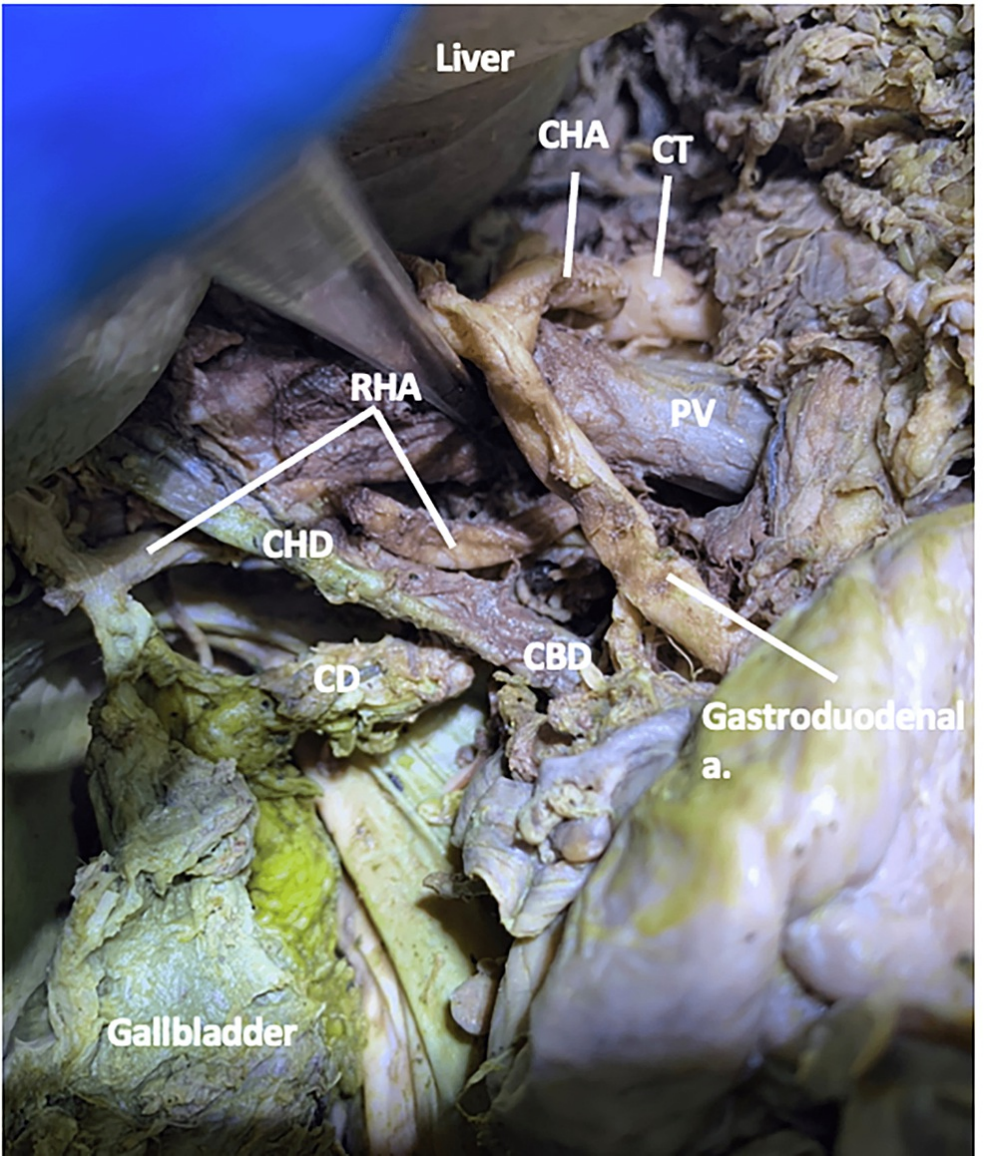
The spatial relationships of the right hepatic artery in case three Case 3: In a 90-year-old female, the right hepatic artery originated from the superior mesenteric artery and traveled deep to the pancreatic neck. The RHA coursed between the portal vein and the proper hepatic artery as it entered the cystic triangle. a: artery; CBD: common bile duct; CD: cystic duct; CHA: common hepatic artery; CT: celiac trunk; PHD: proper hepatic duct; PV: portal vein; RHA: right hepatic artery.
